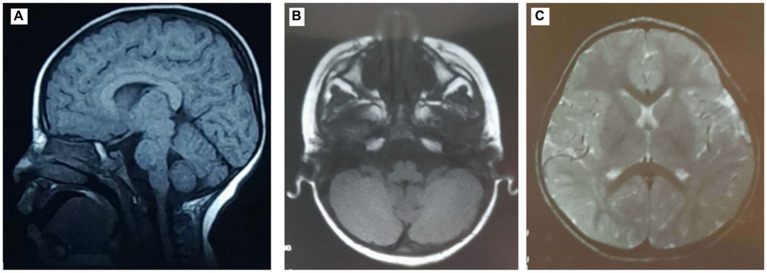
Magnetic resonance images (MRIs) of the patient’s brain showing discrete cerebellum atrophy. (A) Sagittal T1-weighted brain MRI. Axial T1- (B) and T2- (C) weighted brain MRI.
